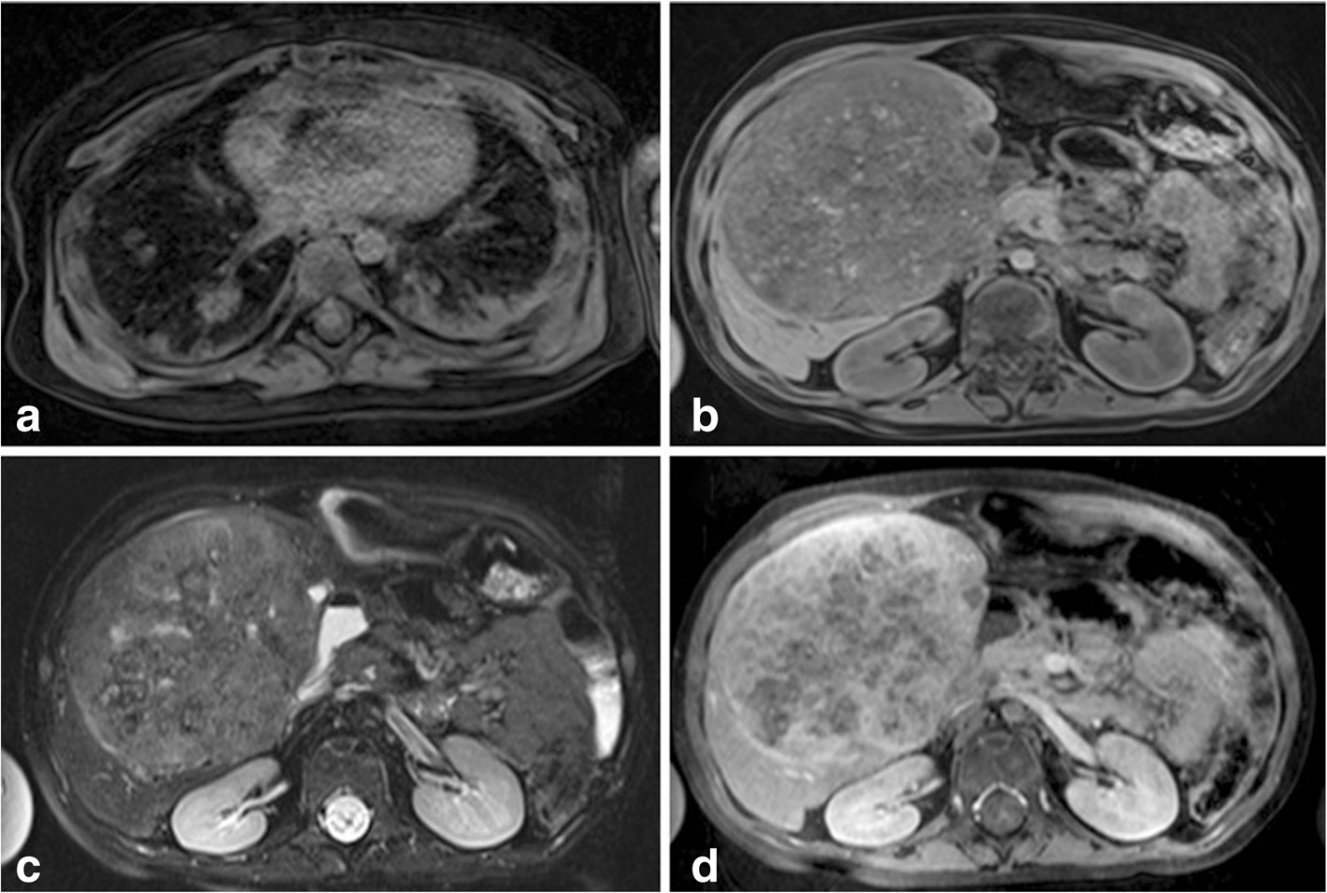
Hepatoblastoma in a 4 year old patient. The (a) axial T1-weighted fat saturated imaging of the lung bases demonstrates multiple pulmonary metastases. On (b) T1-weighted fat-saturated pre-contrast imaging, the hepatic mass has internal heterogenous signal intensity, with overall hypointensity compared to background liver parenchyma. It is mildly hyperintense compared to the liver parenchyma on (c) the T2-weighted fat saturated sequence. On (d) portal venous phase imaging, post gadobenate dimeglumine administration, the lesion has internal heterogeneous enhancement
